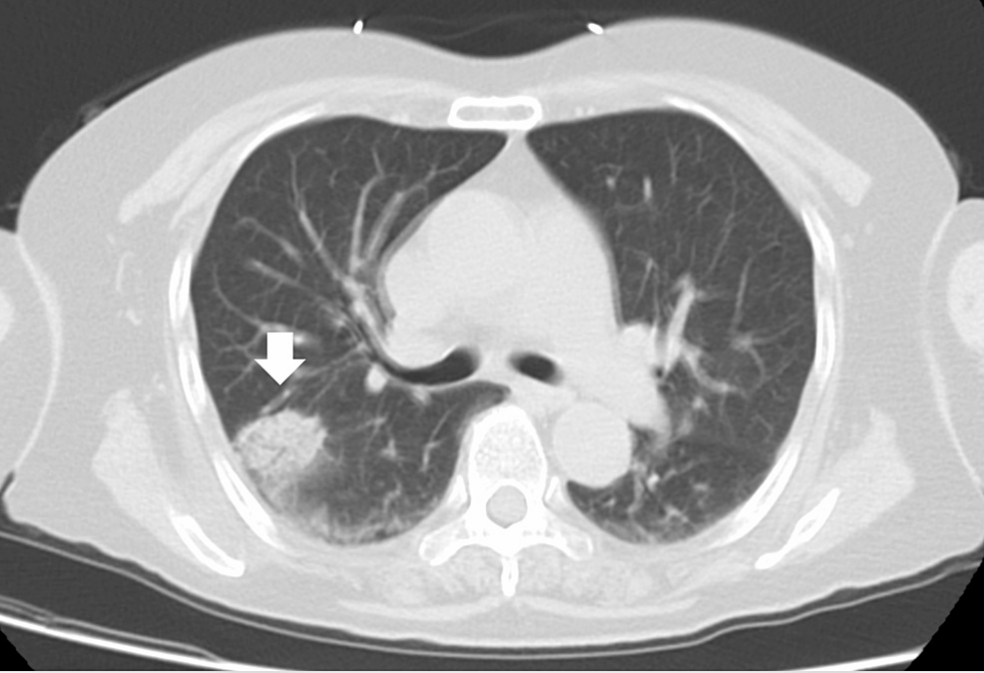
CT scan showed consolidation in the upper lobe of the right lung (white arrow).
